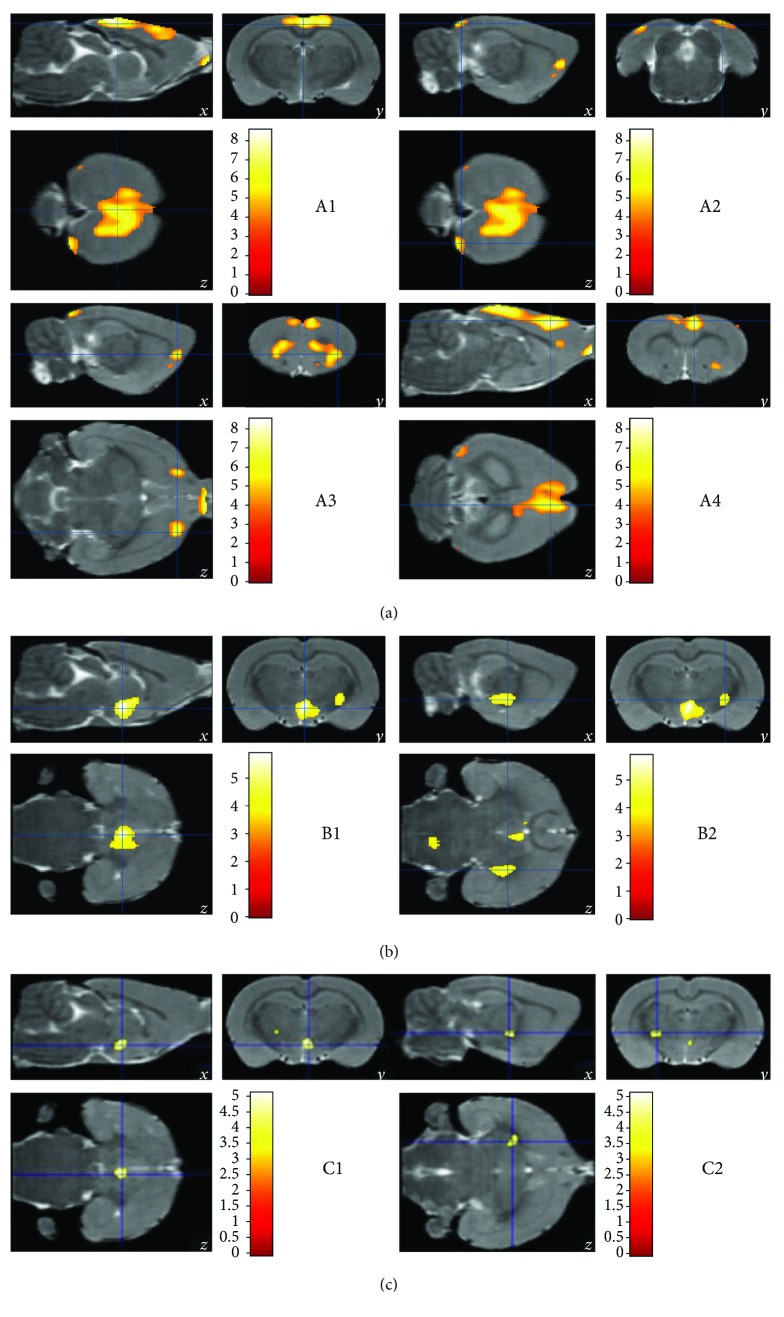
Cerebral areas with decreased (a) or increased (b and c) glucose uptake. The areas with significantly different FDG-PET signals in CG versus MG (a), AG versus MG (b), and NG versus MG (c) (*P* < 0.05) identified in Tables 2, 3, and 4 are shown in these FDG-PET three-dimensional (3D) images. All the data were analyzed with a 2-tailed *t*-test for independent samples.
